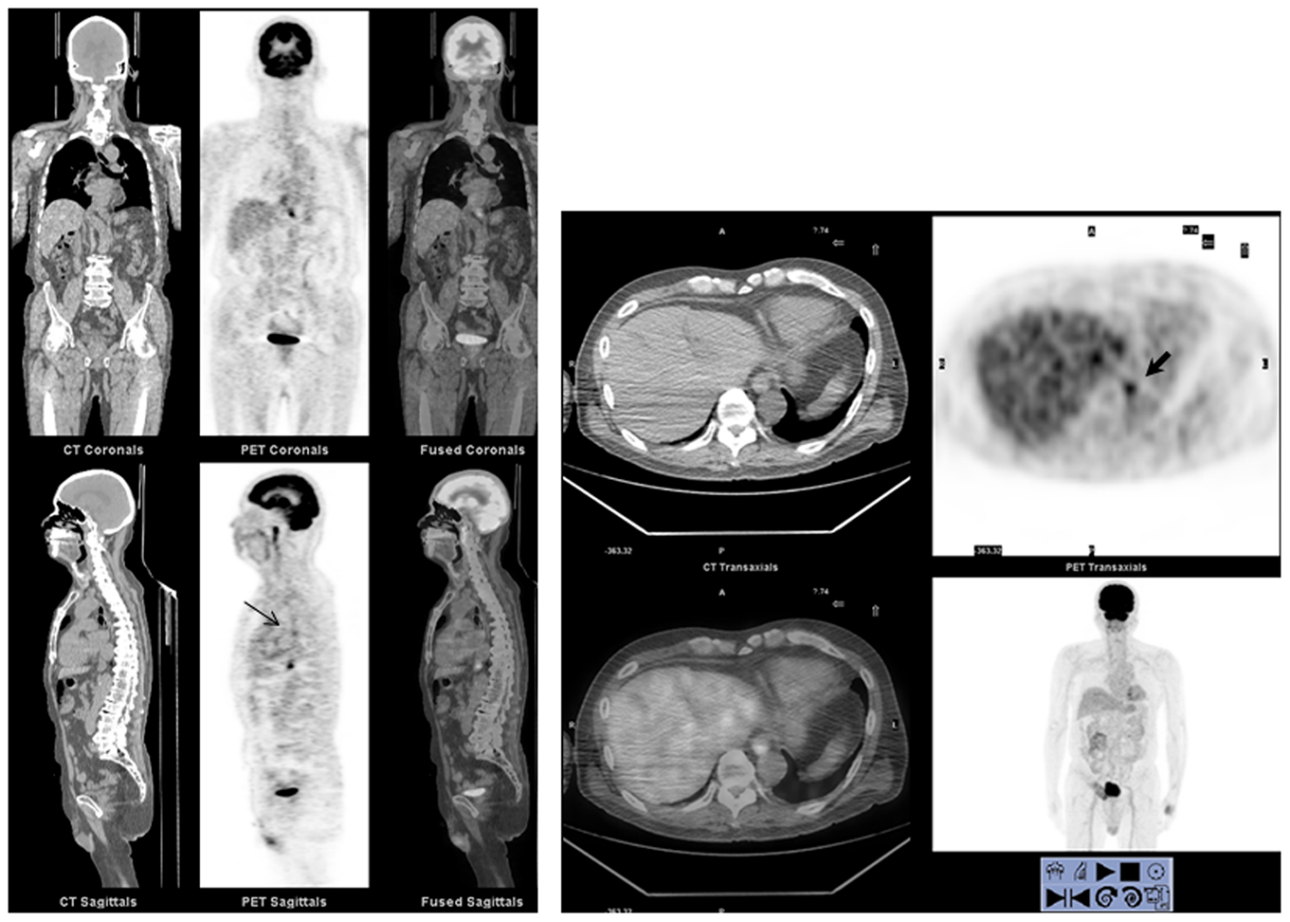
FDG PET/CT images from an 84-year-old male with erosive esophagitis (Los Angeles classification grade A). PET/CT showed two focal areas of FDG accumulation in the middle esophagus (thin arrow on sagittal view, SUV_max_ = 4.5) and at the esophagogastric junction (thick arrows, SUV_max_ = 6.9).
